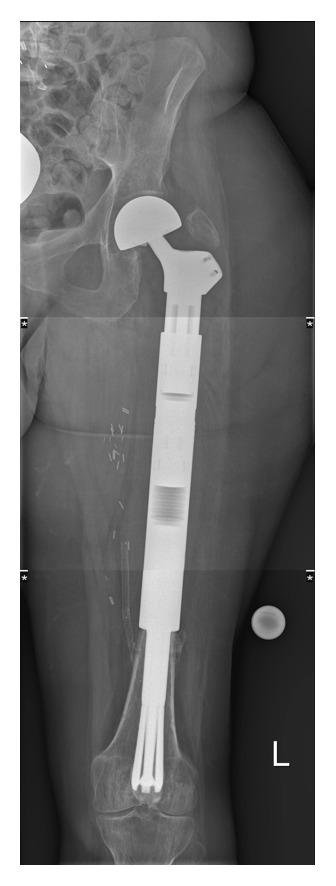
Postoperative X-ray of the implanted spreading stem.
